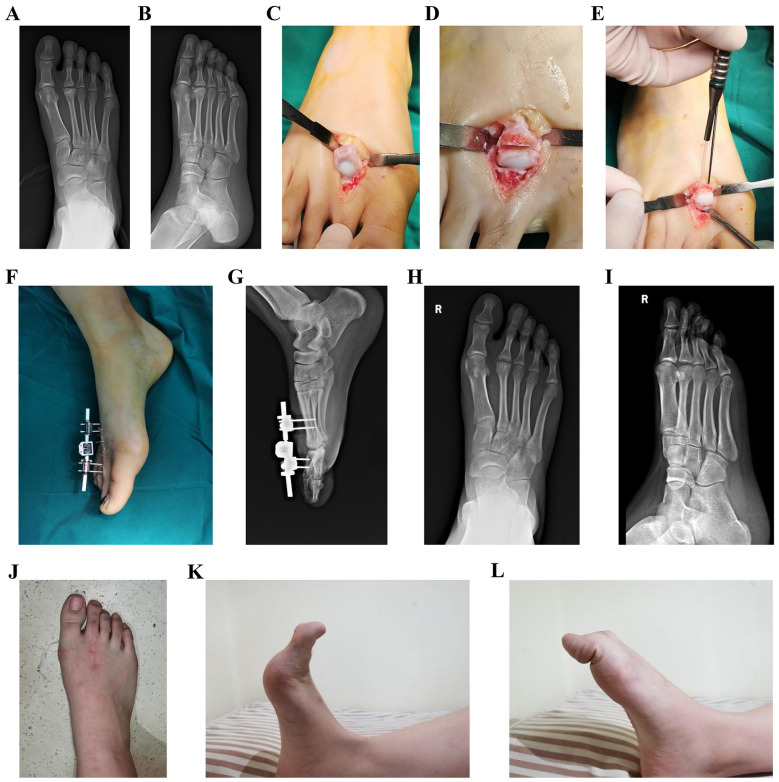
Typical cases. (A,B) Preoperative radiographs; (C–E) surgical procedure; (F) postoperative immobilization; (G) postoperative radiographs; (H–L) radiographs and activity at 28-month postoperative follow-up.
